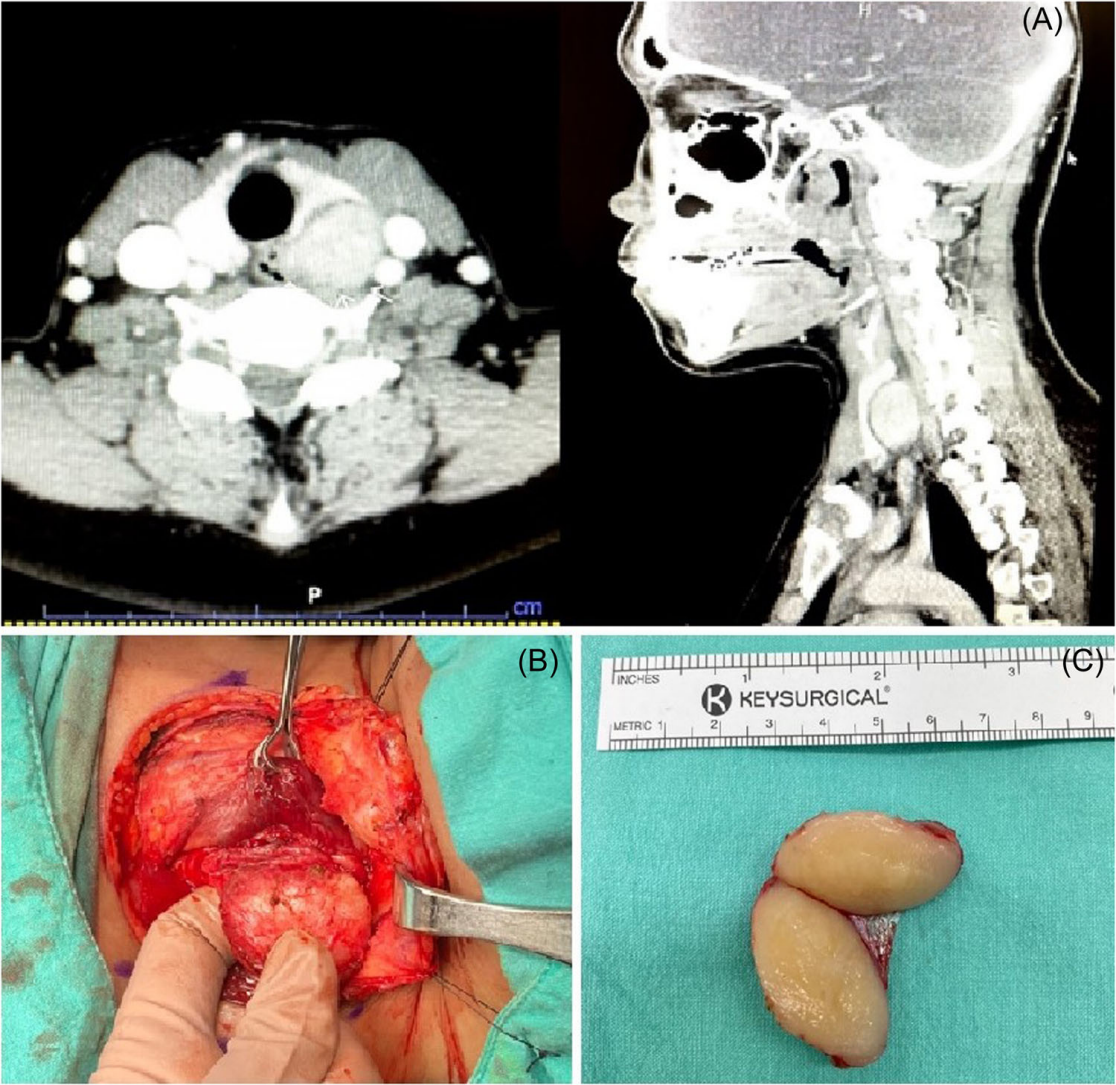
(A) Computed tomography scan showing the mass posterior to the left thyroid lobe with suspicion of esophageal wall involvement. (B) Recurrent laryngeal nerve after thyroid and tumor deflection. (C) Cut surface of the tumor showing an inhomogeneous white‐tan appearance.
